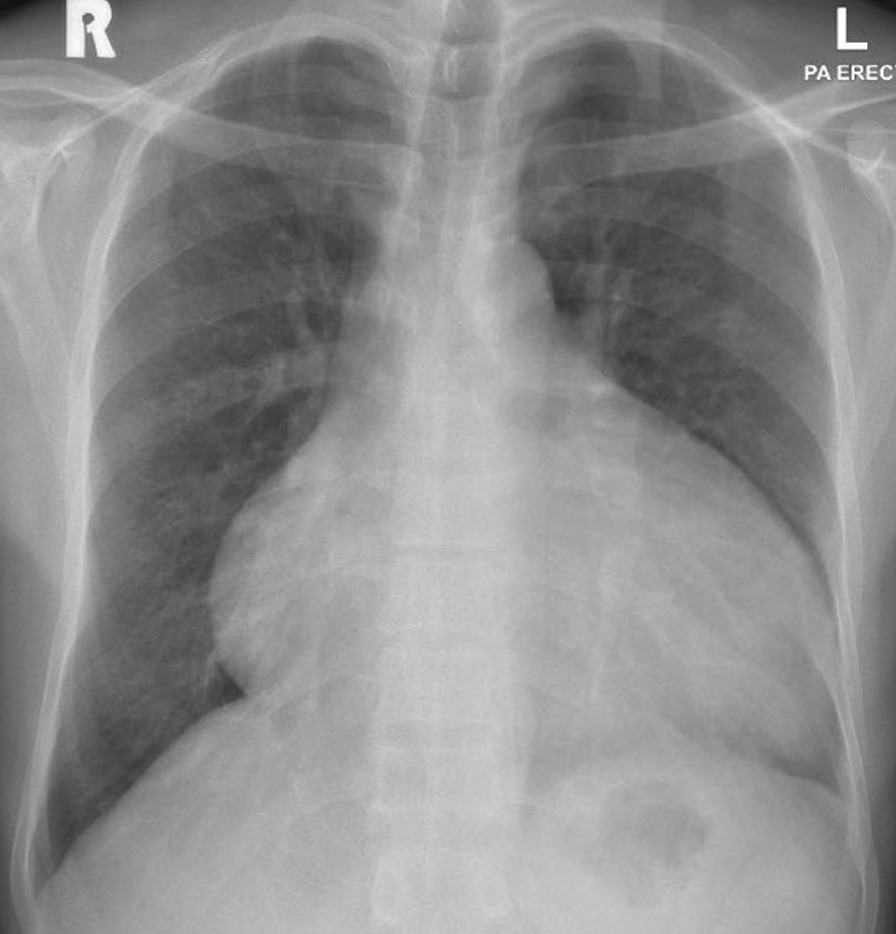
A chest X-ray on admission showed cardiomegaly and pericardial effusion
